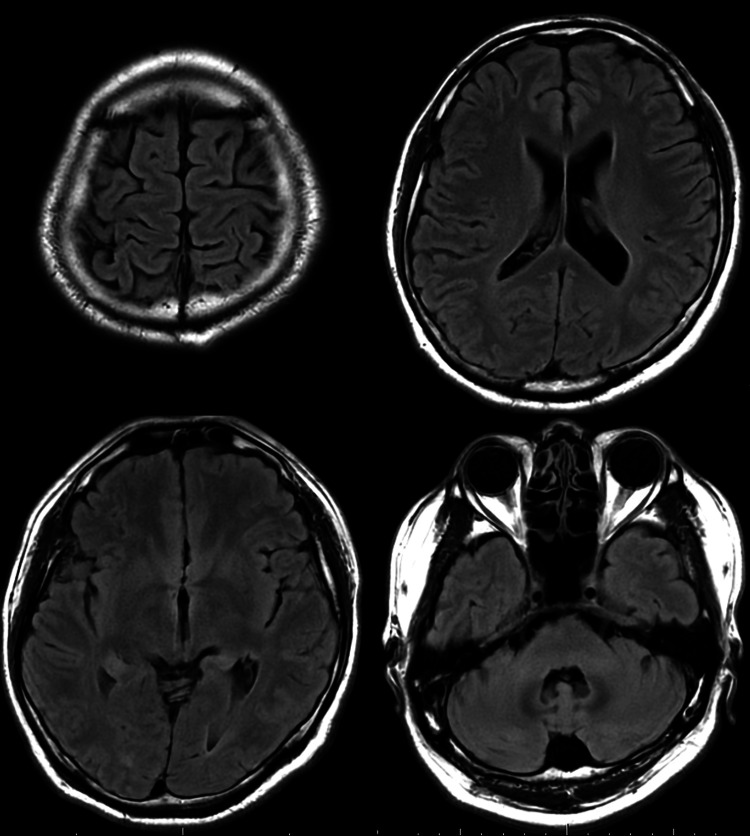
T2-FLAIR brain MRI showing no morphological abnormalities or stroke lesions. T2-FLAIR: T2-weighted fluid-attenuated inversion recovery; MRI: magnetic resonance imaging.
